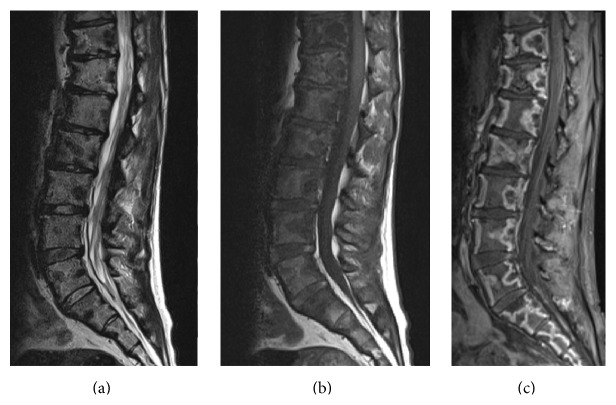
Lumbar spine MRI without and with contrast obtained 6 months after the initial presentation. (a) Sagittal T2 weighted and T1 weighted images without contrast and (c) sagittal postcontrast T1-weighted fat suppressed image. These images show marrow signal alteration with associated band-like areas of enhancement surrounding areas of nonenhancement with associated T1 and T2 hypointensity, consistent with diffuse osteonecrosis.
